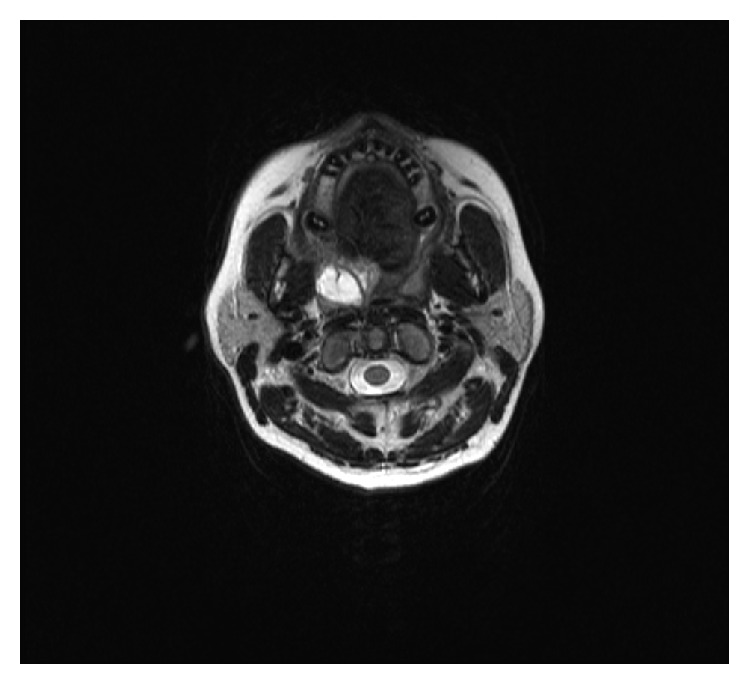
Axial MRI section of the spindle cell lipoma.
